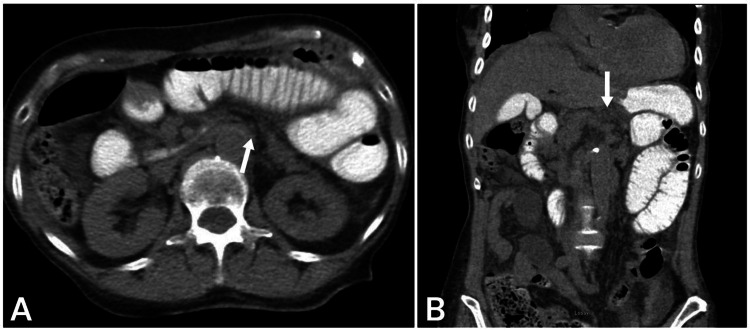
Non-contrast computed tomography (CT). Persistent small bowel distention, with residual contrast from prior imaging confined to the proximal small bowel. A subtle mesenteric rotation is noted in the mid-abdomen (white arrow). (a) Axial view and (b) coronal view.
